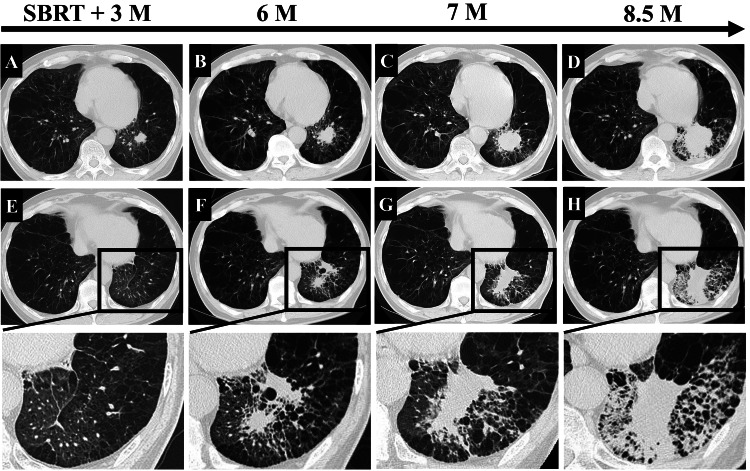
HRCT images after SBRT. (A) HRCT findings showed the reduction of tumors after three months. (B–D) The tumor enlarged gradually again. (E–H) HRCT findings of the development of RILI. Magnified views of the area (bottom) are enclosed by the squares (top). (E) There are no RILI lesions. (F–H) HRCT findings are shown in six months, seven months, and eight and half months after SBRT, respectively. Ground-glass opacities, reticular shadows, and fibrotic changes in the left lower lobe of the irradiated area increased. The development of RILI with fibrotic change was progressive during 8.5 months after SBRT. M, months; HRCT, high-resolution computed tomography; RILI, radiation-induced lung injury; SBRT, stereotactic body radiation therapy.
